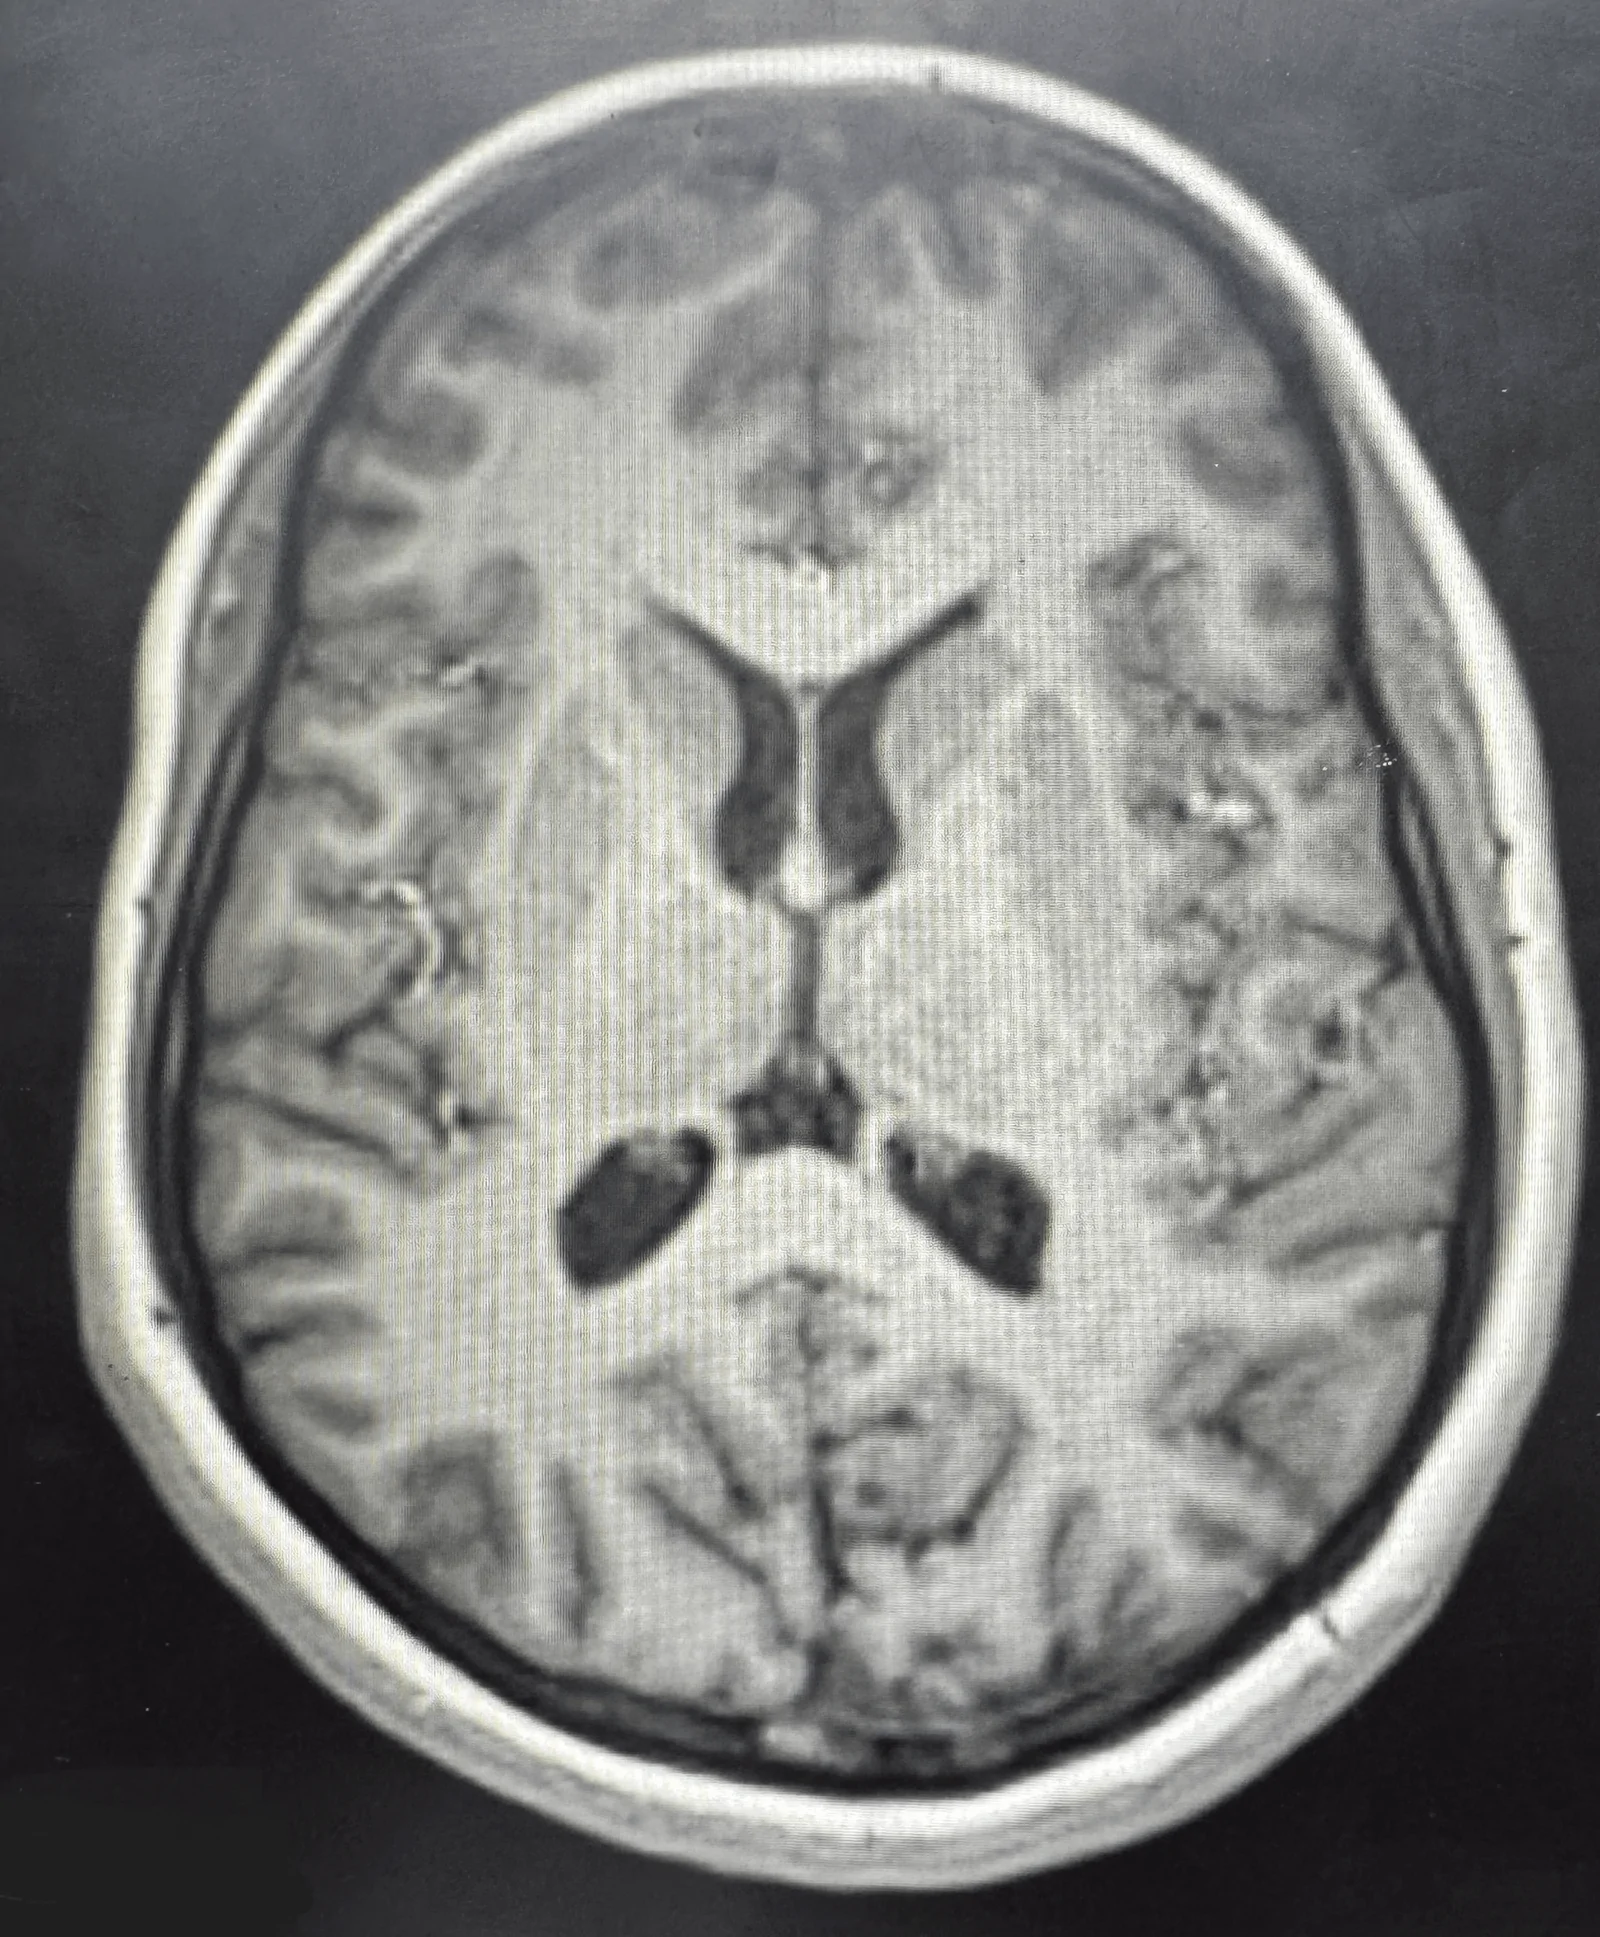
Axial T1-weighted brain MRI showing no structural brain abnormality. Gray-white matter differentiation, ventricular size, and cerebral parenchyma are preserved, with no evidence of focal lesions, cerebral atrophy, or hydrocephalus.
